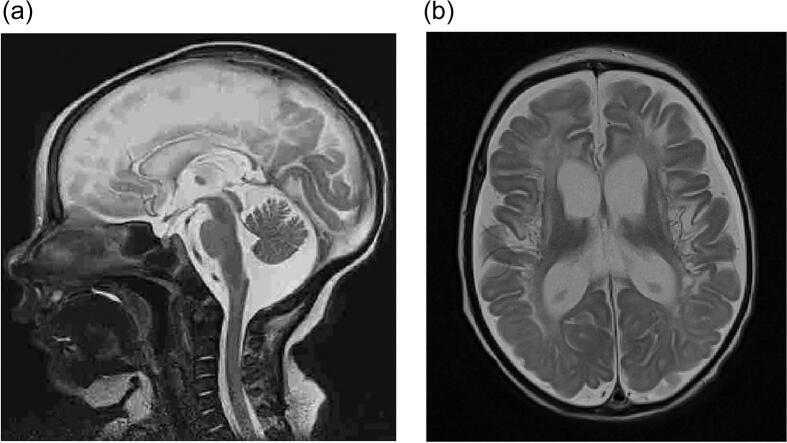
a MRI on day 8 of life - picture of diffuse cytotoxic edema, no signs of bleeding. b MRI at five weeks of age generalized atrophic changes in both brain hemispheres. Broad cisterns of the basal of the brain. T1 -T1-weighted image shows abnormal high signal intensity in frontal and parietal lobes, central furrows, subcortical islands, and basal ganglia. The white matter of both brain hemispheres has decreased volume and abnormal signal intensity, hyperintense on T2-weighted images, and hypointense on T1-weighted images, with cavities forming.
